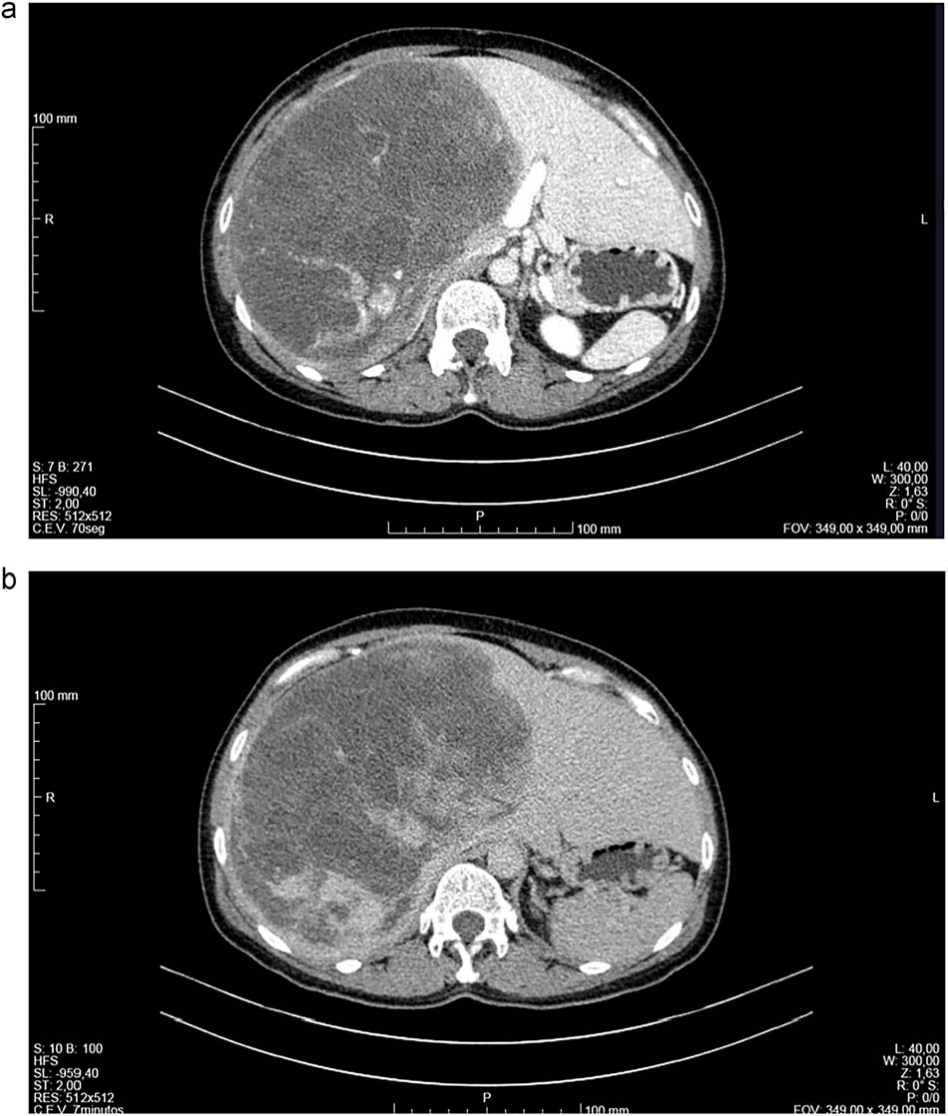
(a, b) Computed tomography (CT) scans of a woman aged 49 years with undifferentiated embryonal sarcoma of the liver. Liver of increased dimensions due to the presence of voluminous oval mass and well-defined limits occupying practically the entire right lobe (measures 16 × 16 × 20 cm^3^). It is a heterogeneous mass, with areas of apparently liquid spontaneous density. Captures contrast gradually and progressively.
